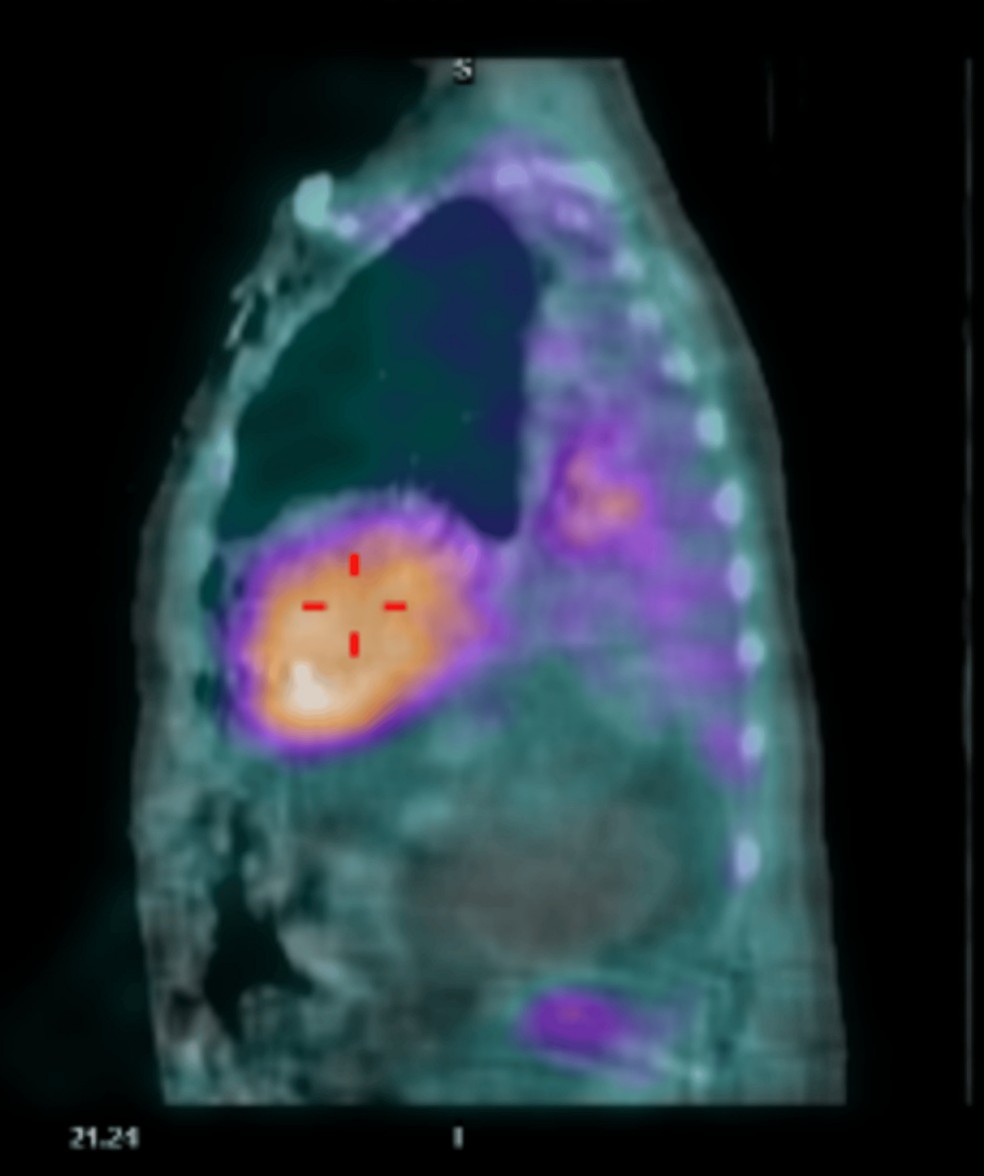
Sagittal projection of fused SPECT/CT images displaying mild Tc99m PYP uptake in the right ventricular free wall SPECT/CT images acquired 60 minutes after the injection of radiotracer. SPECT: Single-photon emission computed tomography; Tc99m PYP: technetium pyrophosphate
